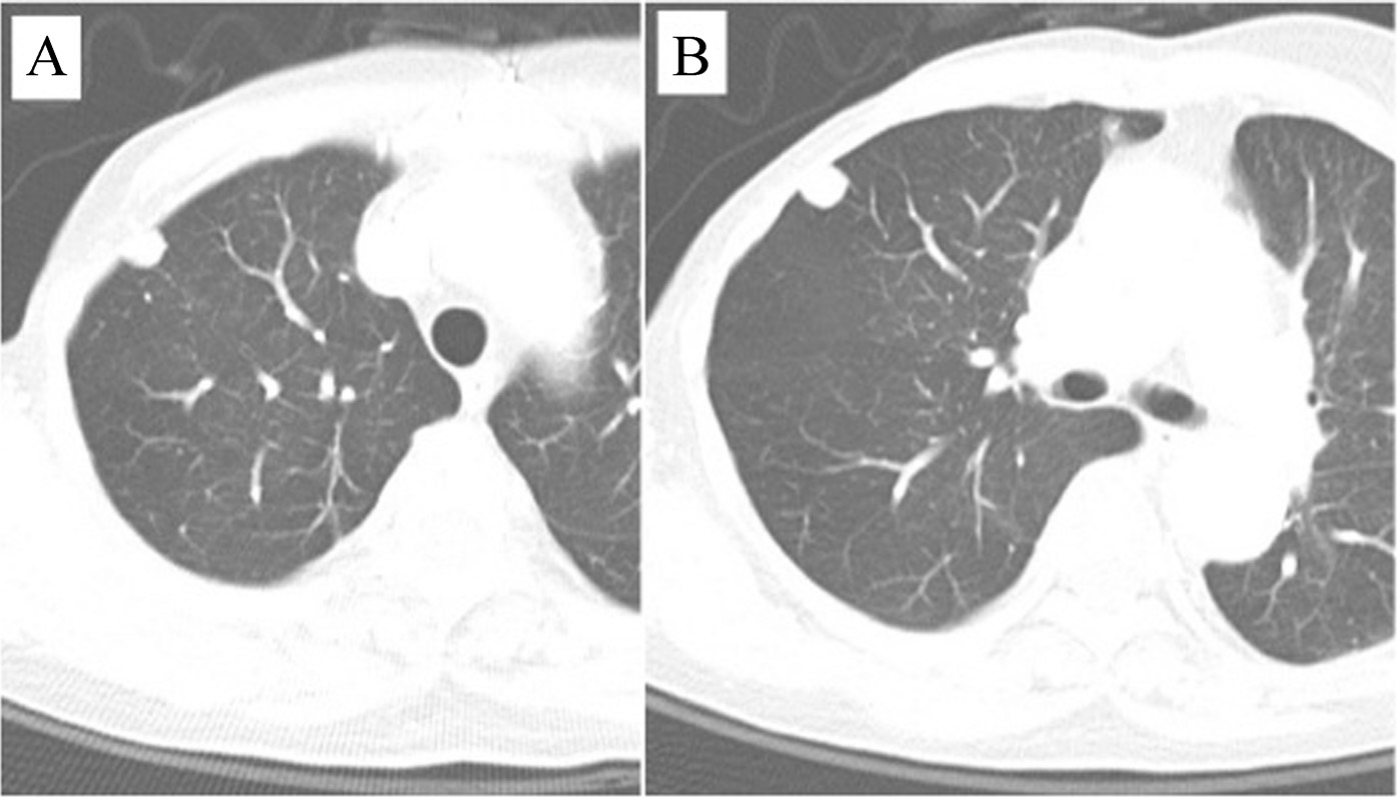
Chest CT of case 2 shows a nodular shadow in the right S1 (a) and in the right S3 (b)
